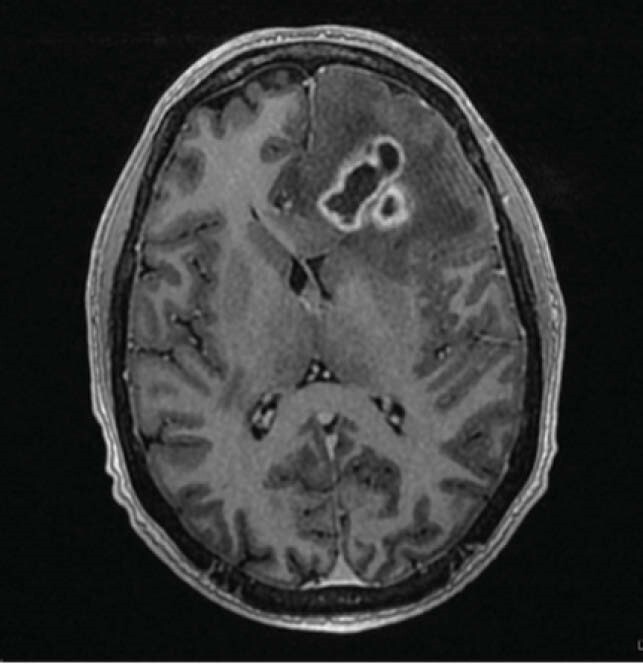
Head CT s/p left frontal craniotomy with resection & evacuation of abscess
